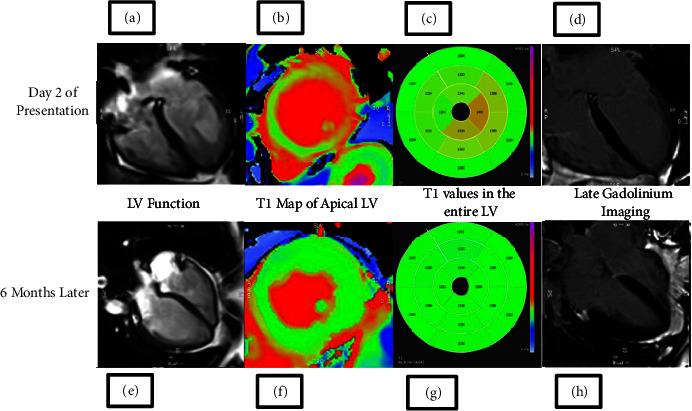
Cardiovascular Magnetic Resonance imaging (CMR) of case 3 performed just prior to the coronary angiogram showed classical features of TCM. There was severe hypokinesia (a) and evidence of myocardial oedema on T1 map (b and c) of mid to apical regions of the left ventricle. The LV ejection fraction was moderately impaired at 43% and the mean global T1 time was elevated at 1311 ms. Normal T1 time for a female in our 3 Tesla scanner is 1151–1251 ms. Late Gadolinium Enhancement (LGE) imaging confirmed the absence of myocardial infarction or fibrosis (d). CMR at 6 months shows complete resolution of the regional wall motion abnormalities (e) and myocardial oedema (f and g). The LVEF was much improved to 56% and the mean global T1 time became normalised to 1192 ms with no significant regional differences. Once again, there was no evidence of any myocardial infarction nor fibrosis on LGE imaging (h).
